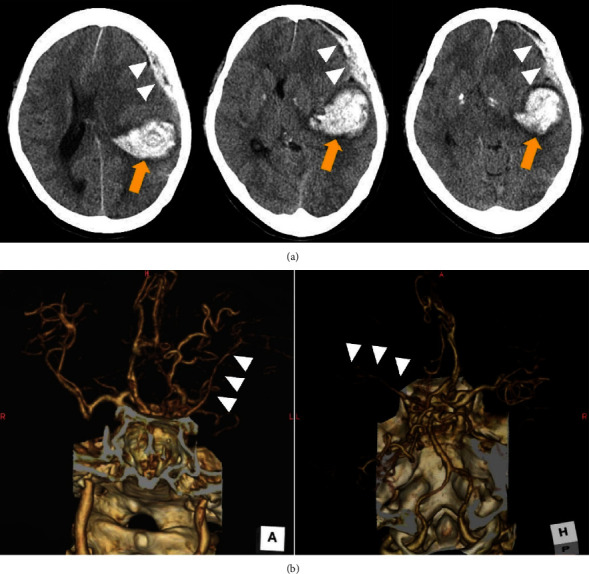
Preoperative radiological examinations. (a) Computed tomography (CT) showed subcortical temporoparietal hemorrhage (arrow) and subdural hematoma (arrowheads) with midline shift of the cerebrum as well as uncal herniation. (b) Three-dimensional CT angiography indicated scarcely enhanced the left middle cerebral artery (arrow heads): (left) anterior-posterior and (right) posterior-anterior view.
